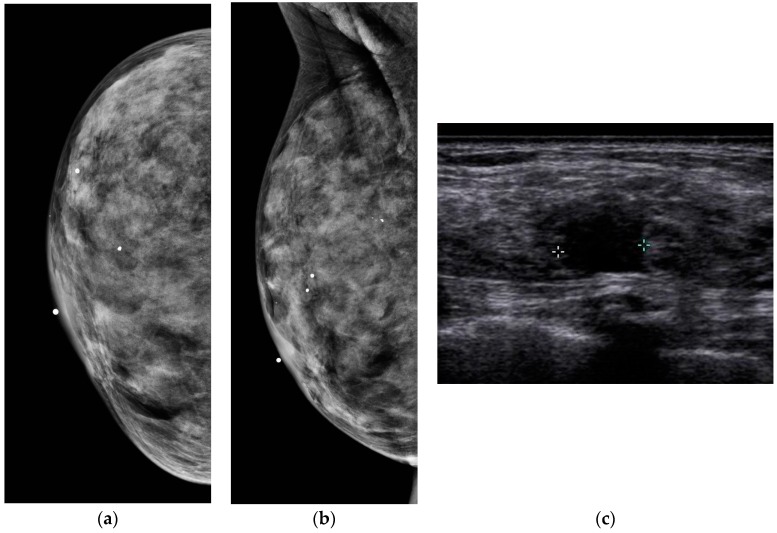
A 43-year-old woman with extremely dense breast tissue. Dense tissue obscures a breast cancer that is easily visible with ultrasound imaging. (a,b) Craniocaudal (CC) and mediolateral oblique (MLO) digital mammography. (c) Handheld high-resolution ultrasound demonstrates a 1.2 cm irregular mass, denoted by calipers in the image, which was biopsied and proven to be invasive ductal carcinoma.
